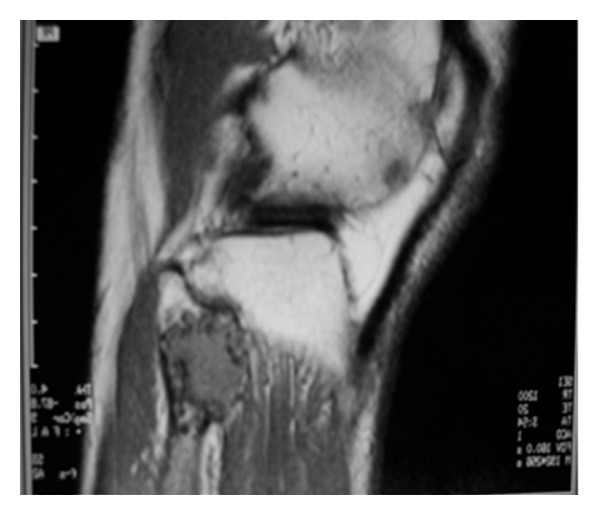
MRI of the same lesion showing cortical damage and soft tissue mass.
